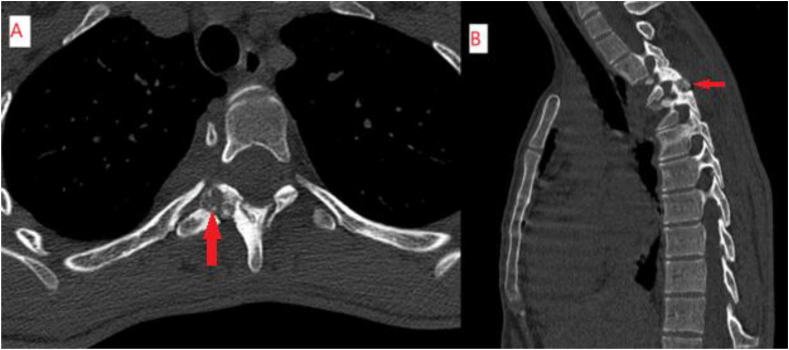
Computed tomography (CT) of the thoracic spine showing a well-defined lytic lesion (10 × 11 mm; red arrows) predominantly involving the right T4-5 facet joint, with extension toward the origin of the right T5 transverse process, consistent with an osteoblastoma pattern. (A: axial cut; B: sagittal cut).
